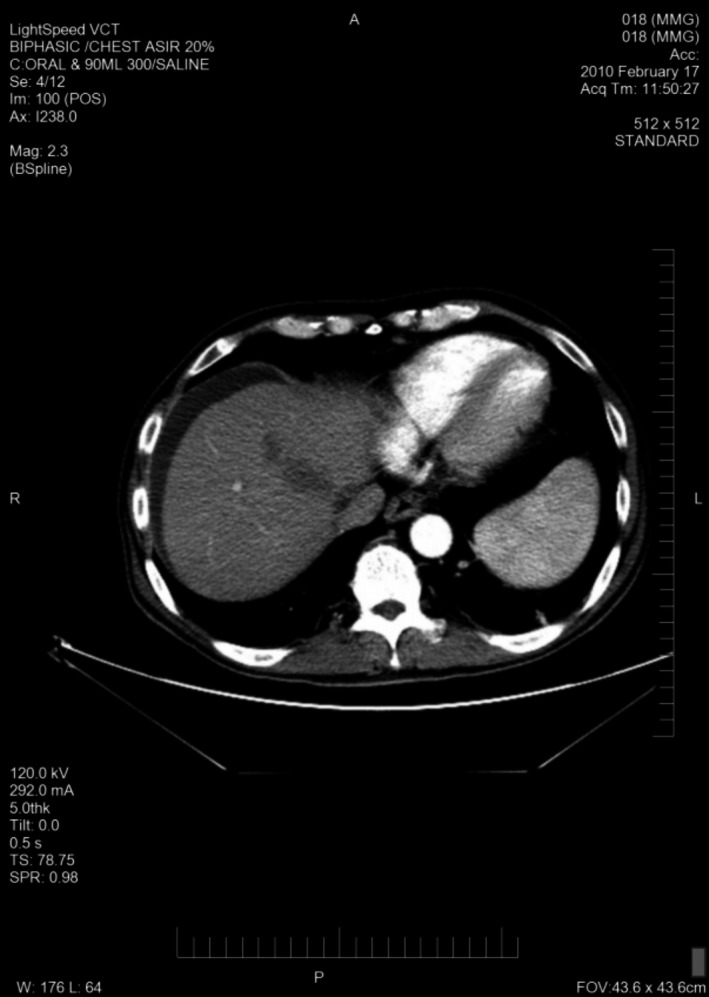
Patient 1 with Hepatocellular Carcinoma (HCC), 6‐month follow‐up CT arterial phase.
